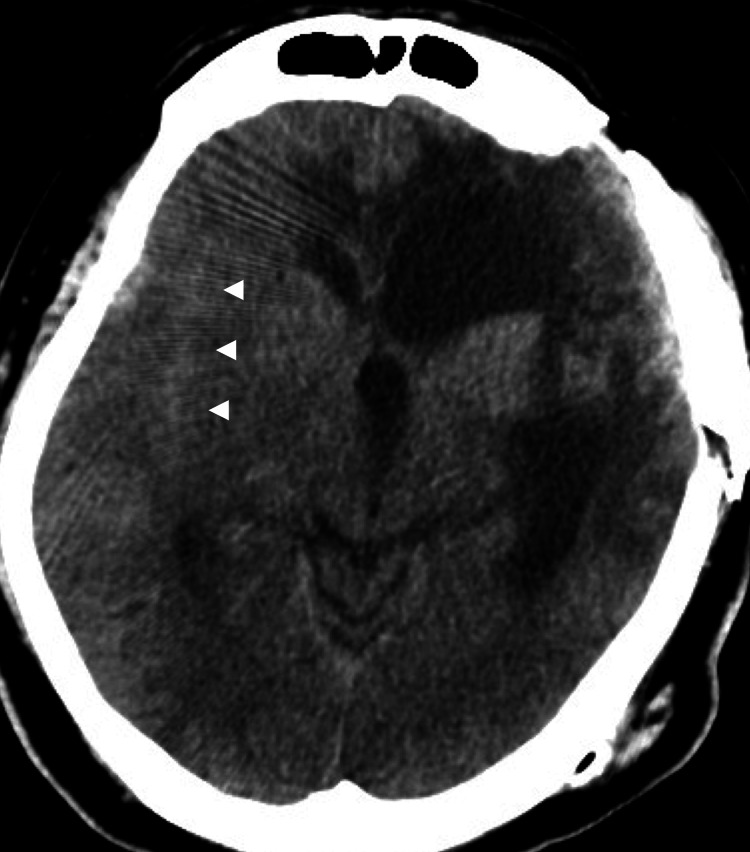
Non-contrast computed tomography (CT) on admission Axial non-contrast CT scan showing high-density areas within the right Sylvian fissure (arrowheads), consistent with a subarachnoid hemorrhage (Fisher grade 2).
